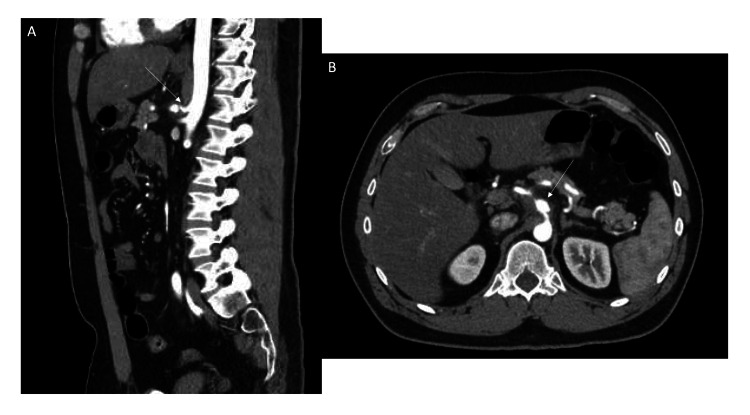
CTA of the abdomen and pelvis at 48 hours. A) Sagittal view shows persistent compression of the celiac artery by the median arcuate ligament (white arrow), with a stable aneurysm of the celiac artery.
